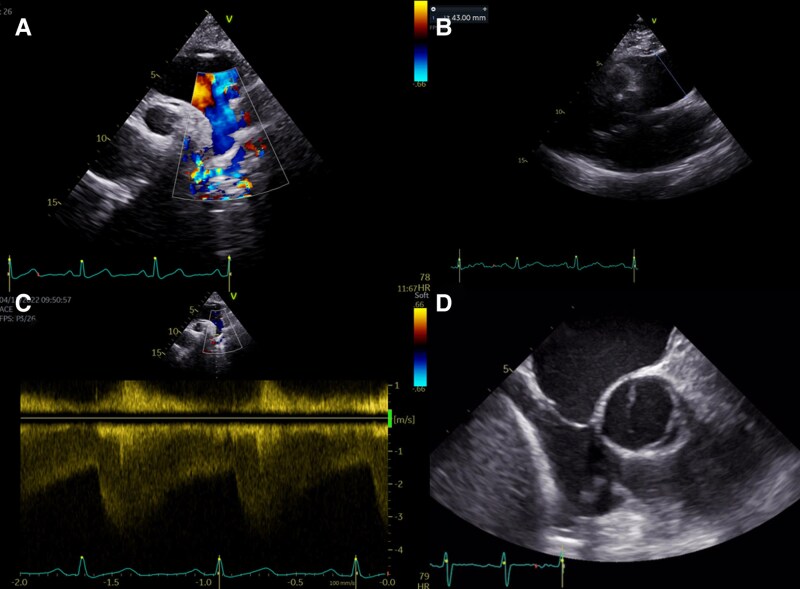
Echocardiographic findings. *(A*) Suprasternal window echocardiogram revealing turbulent flow in the descending thoracic aorta. *(B*) Transthoracic echocardiogram demonstrates a dilated ascending aorta measuring 43 mm. *(C*) Continuous Wave Doppler at the coarctation site exhibiting the characteristic ‘sawtooth’ pattern. *(D*) Transoesophageal echocardiogram reveals a Siervers type 1 bicuspid aorta.
